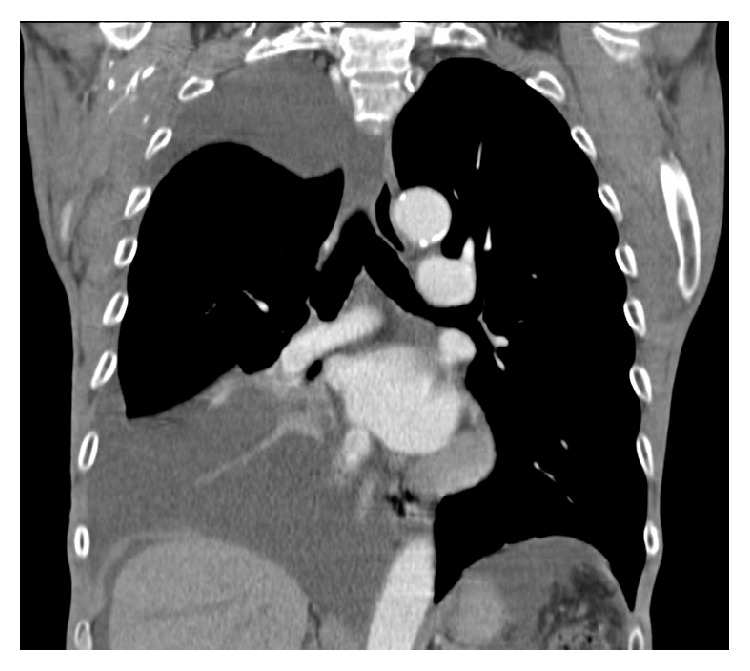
CT scan of the chest with intravenous contrast (coronal view) showing large right sided pleural effusion with lower lobe atelectasis and perihepatic ascites.
